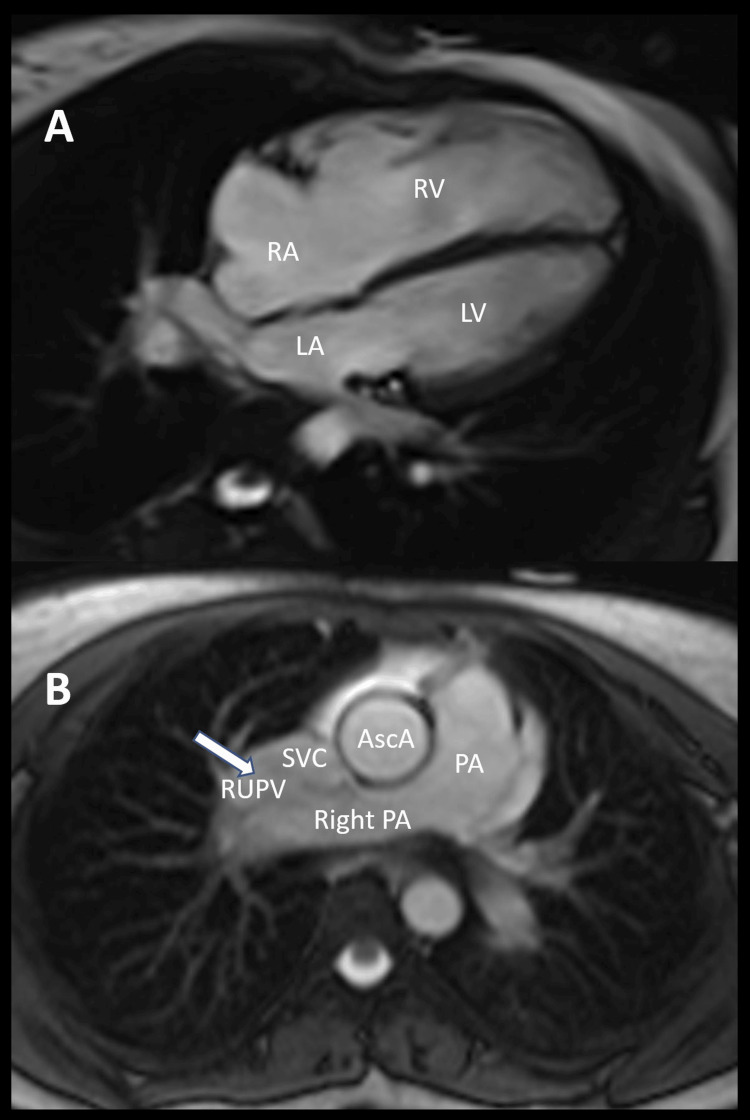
Cardiac MRI revealing partial anomalous pulmonary venous return. (A) Still image from a four-chamber cine showing significant right ventricular (RV) dilation with the right atrium (RA), left atrium (LA), and left ventricle (LV) labeled, respectively. (B) Axial image of partial anomalous pulmonary venous return (arrow) from the right upper pulmonary vein (RUPV) into the superior vena cava (SVC). Main pulmonary artery (PA) is dilated. Ascending aorta (AscA) and right pulmonary artery (PA) are labeled.
